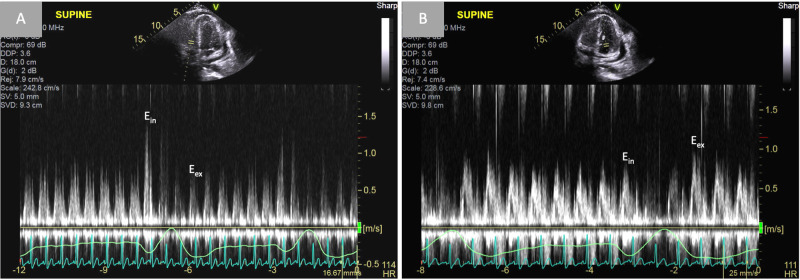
Demonstration of constrictive pathophysiology via tricuspid and mitral inflow velocities with respiratory variation Pulse-wave Doppler findings from the tricuspid (A) and mitral (B) inflow velocities with simultaneous respirometric recording (green line). Greater than 40% respiratory variation in the peak tricuspid E inflow velocity and greater than 25% respiratory variation in the peak mitral E inflow velocity are consistent with constrictive physiology. E_in_ and E_ex_ represent E velocity during inspiration and expiration, respectively.
